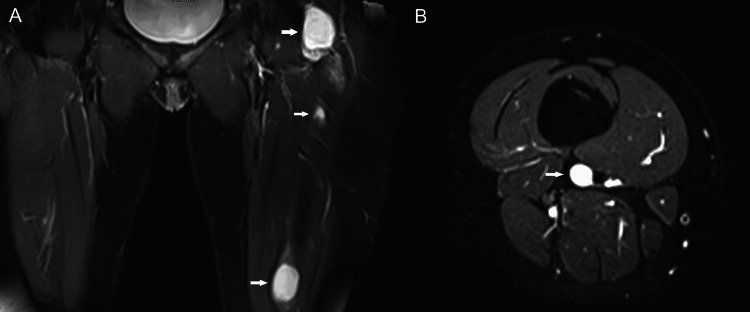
Coronal (A) and axial (B) STIR MR images depict myxomas (arrows) of high signal intensity in musculature of the left thigh.
